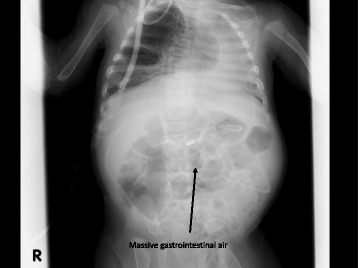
A chest-abdominal X-ray at deteriorated respiratory status. A subsequent chest-abdominal X-ray revealed that gastrointestinal air was severely accumulated
